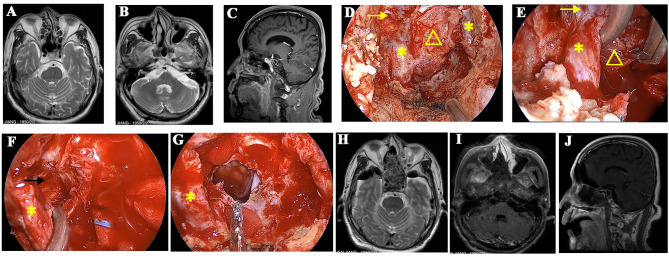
A recurrent chordoma locates in the upper and mid-clivus. (A, B) The axial MR images show that the tumor locates in the right posterior space of cavernous ICA and the right posterior space of paraclival ICA, respectively. (C) The sagittal view shows that the tumor locates in the upper and mid-clivus and invades into the posterior space of paraclival ICA. (D) Intraoperative image shows that the bone covering the paraclival and lacerum ICA was drilled. (E) The paraclival ICA is gently retracted, and the tumor behind ICA was resected with the angled instruments under the view of 45° endoscopy. (F) The posterior space of cavernous ICA is seen after tumor resection, and the margin is clear. (G) The tumor is totally removed, and brain stem is visible. (H–J) Postoperative MR images showed that the tumor was totally removed. The yellow arrows represent cavernous ICA. The yellow triangles represent the recurrent chordoma. The yellow asterisks represent paraclival ICA. The black arrow represents the posterior space of the cavernous ICA.
